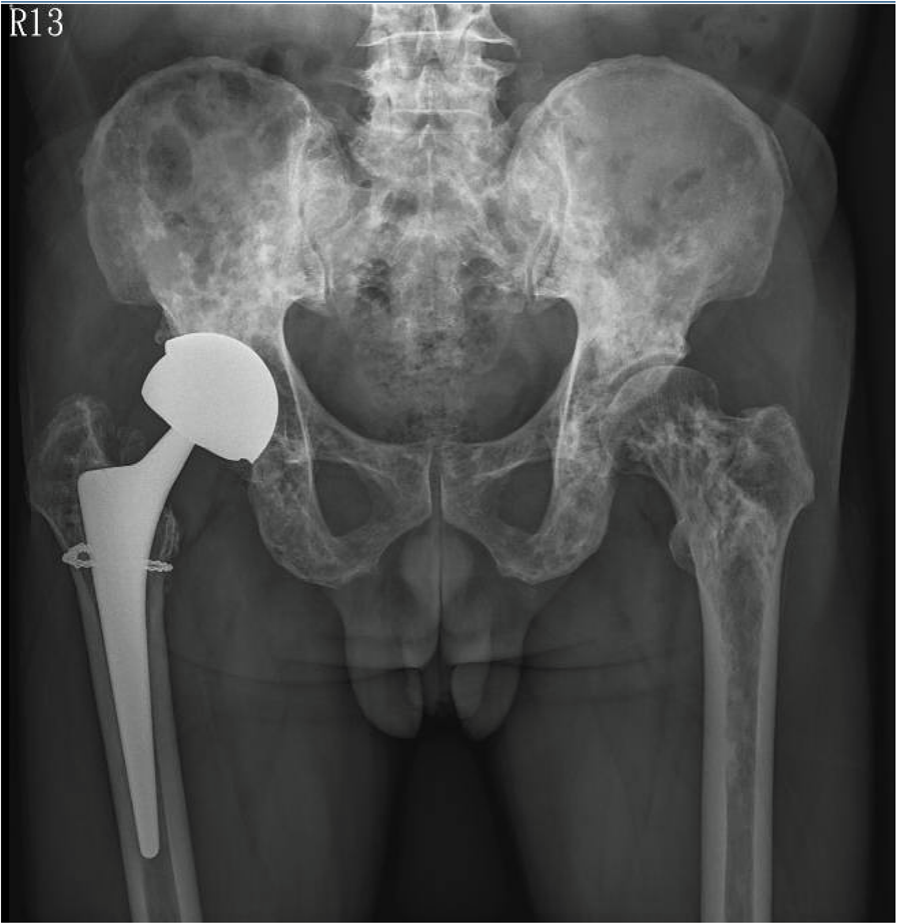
Plain radiography of pelvis. Well-fixed prosthetic components
